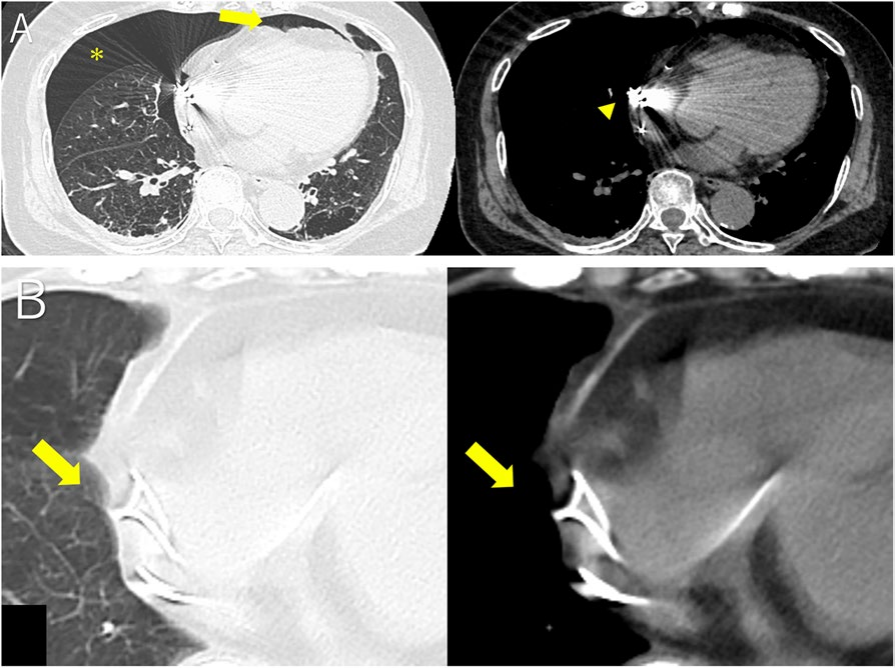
Computed tomography images. A The asterisk (✻) indicates the area of the collapsed lungs. The yellow arrow indicates pericardial emphysema. The yellow arrowhead indicates the tip of the lead. The tip appears to be outside the myocardial wall. B Postoperative computed tomography image. Pneumothorax has not recurred. The yellow arrows indicate residual adipose tissue at the tip of the right atrial lead
